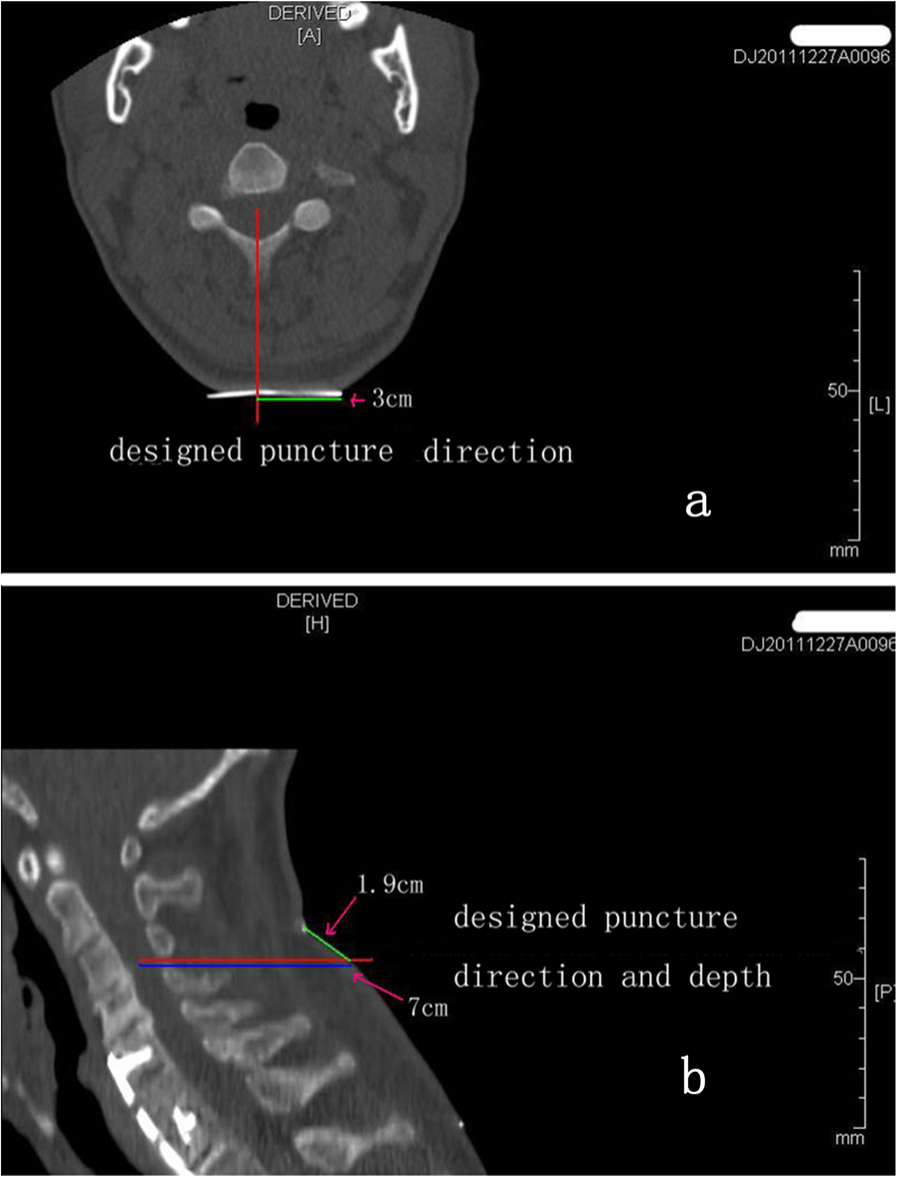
CT machine ascertainment of the intended cell puncture site, direction and depth. a, An axial (transverse) image. The red line indicates the upper intervertebral space of the intended puncture site and the direction. The green line shows that the intended puncture site is 3 cm away from the left edge of the marker. b, A sagittal image. The pink line represents the upper intervertebral space of the intended puncture site and the direction. The blue line shows the intended puncture depth of 7 cm, and the green line shows that the intended puncture site is located 1.9 cm below the upper marker.
